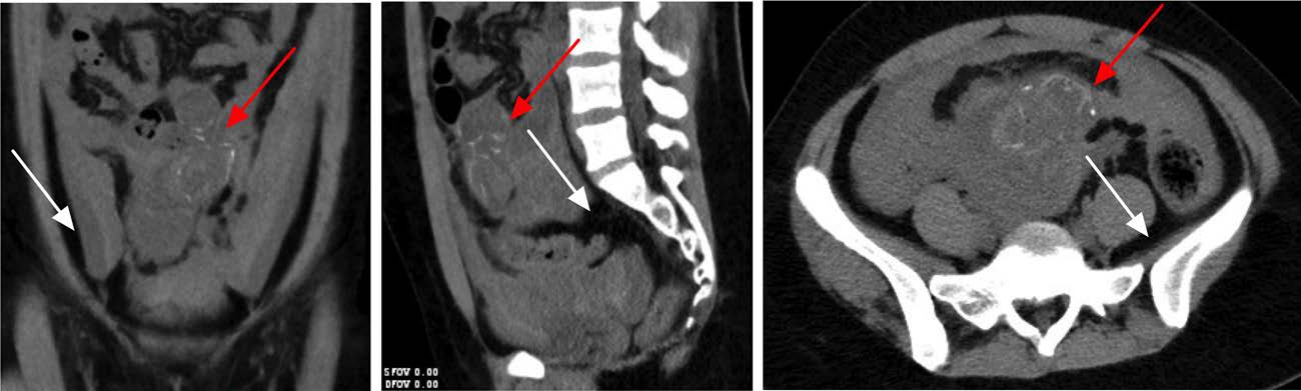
Preoperative computed tomography scan of the abdomen with arrow marks showing fetal mass (red) and bloody effusions (white).
